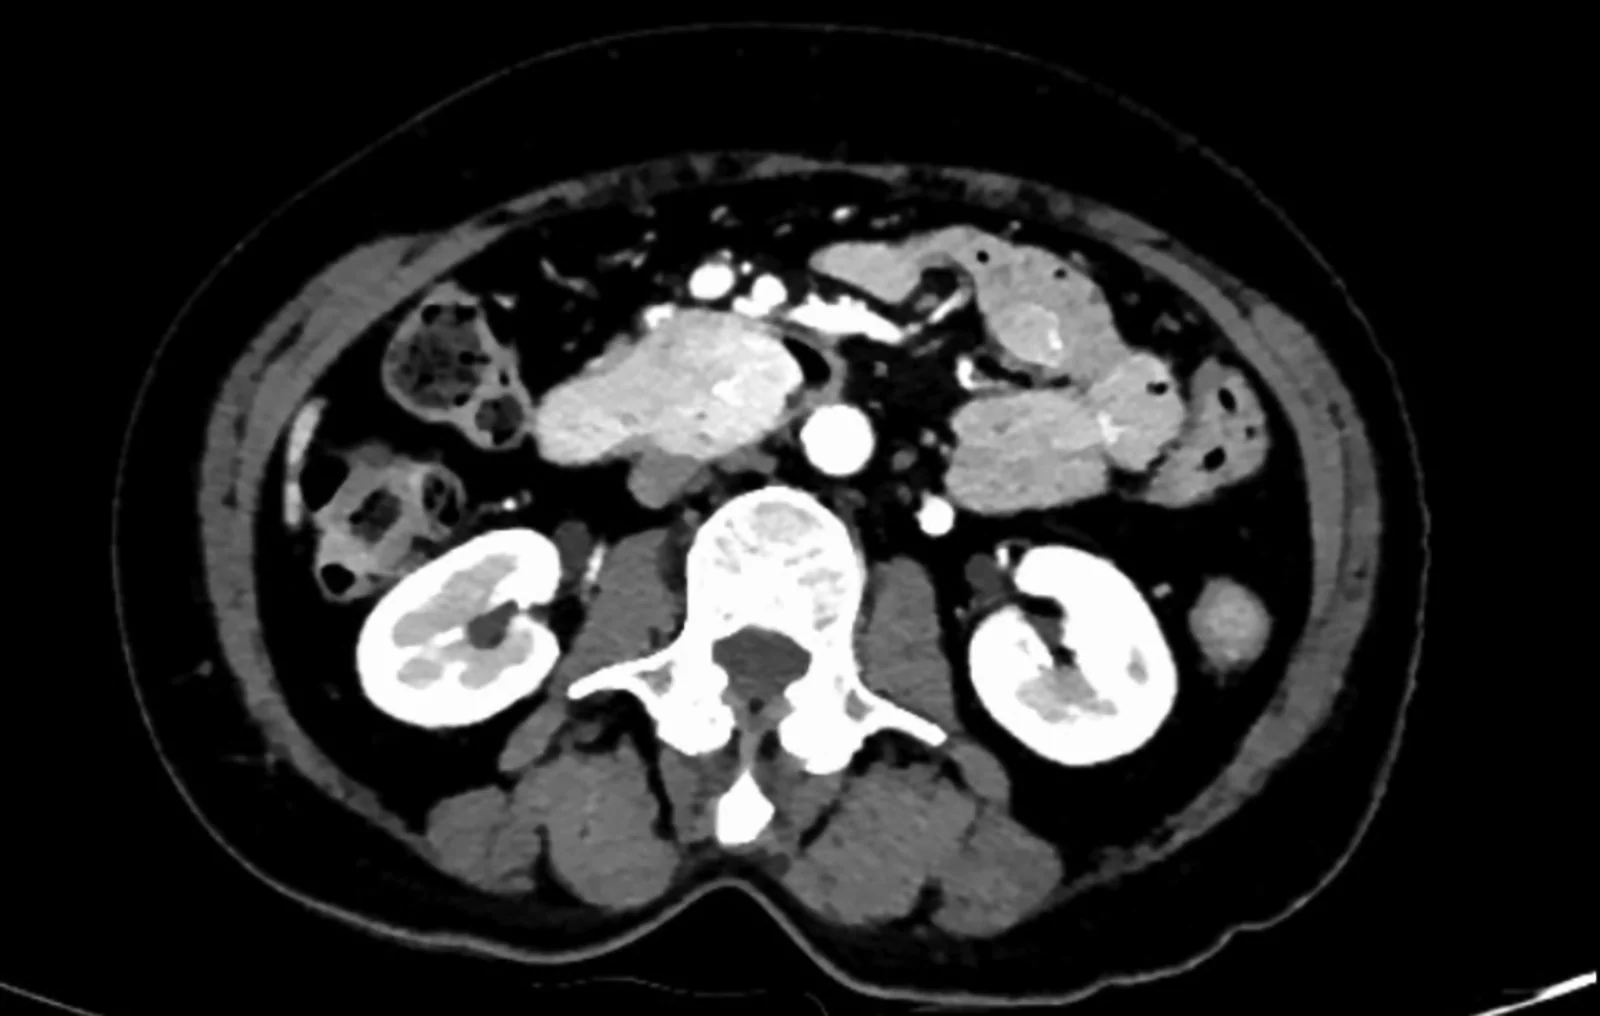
Abdominal enhanced CT shows a space-occupying lesion at the descending and horizontal parts of the duodenum; the lesion exhibits homogeneous enhancement on enhancement scan, with no enlarged lymph nodes in the periphery.
